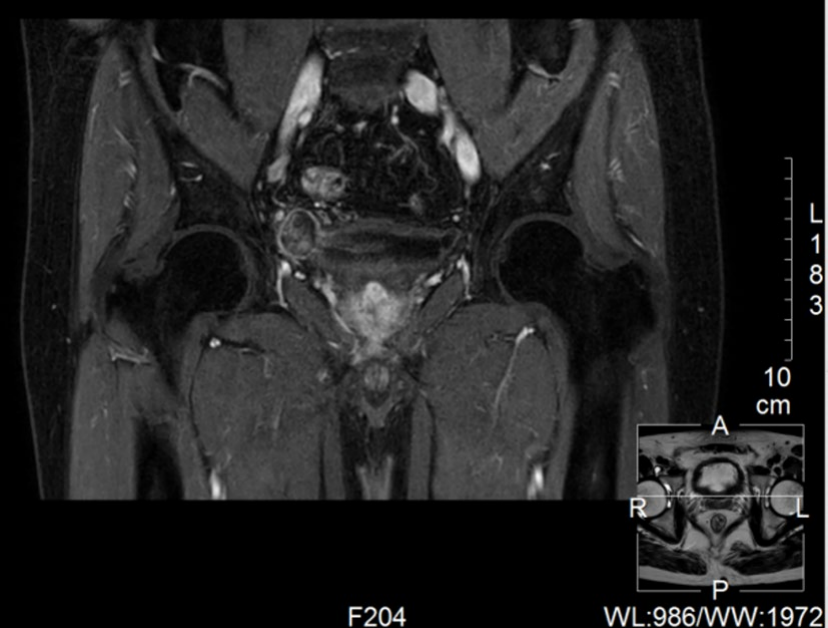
Magnetic Resonance Imaging (MRI) scan reveals a right lateral wall diverticulum of the bladder with a space-occupying lesion scan.
